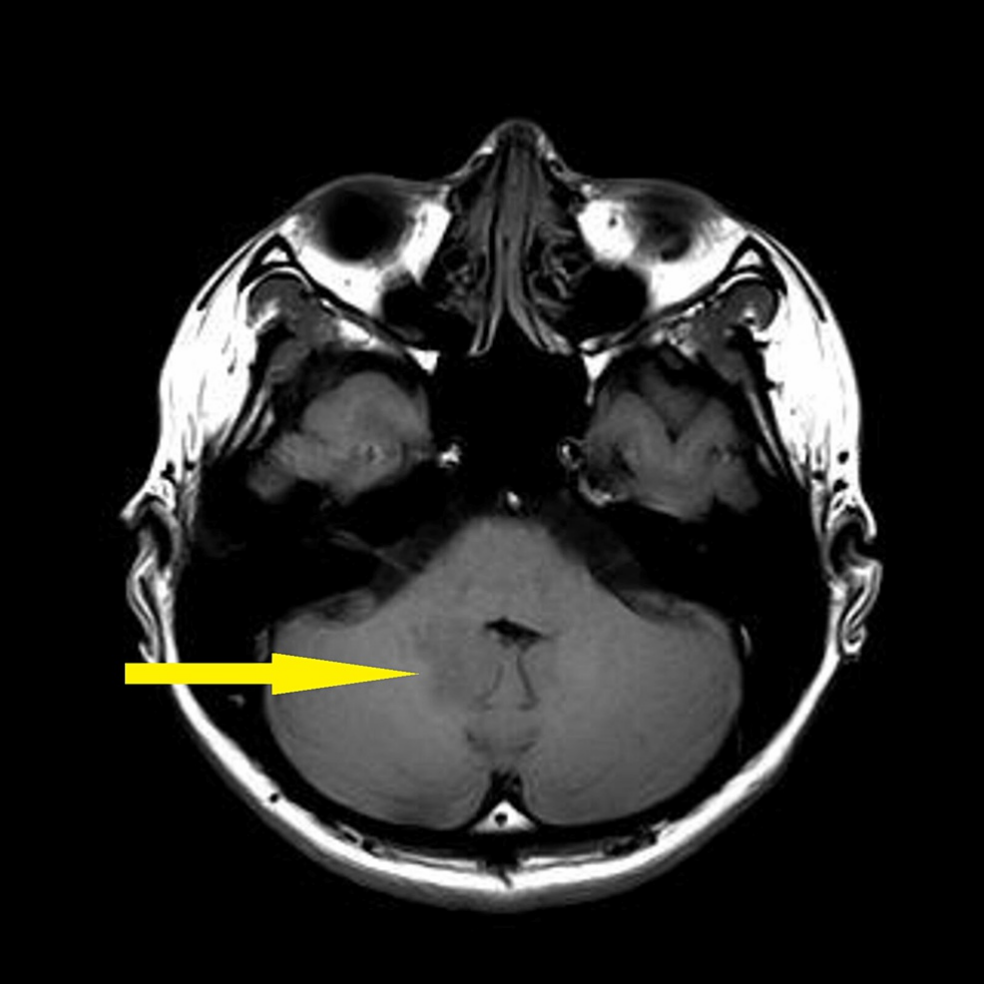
MRI brain (T1) axial showing an ill-defined lesion in the fourth ventricle, appearing isointense (yellow arrow)
